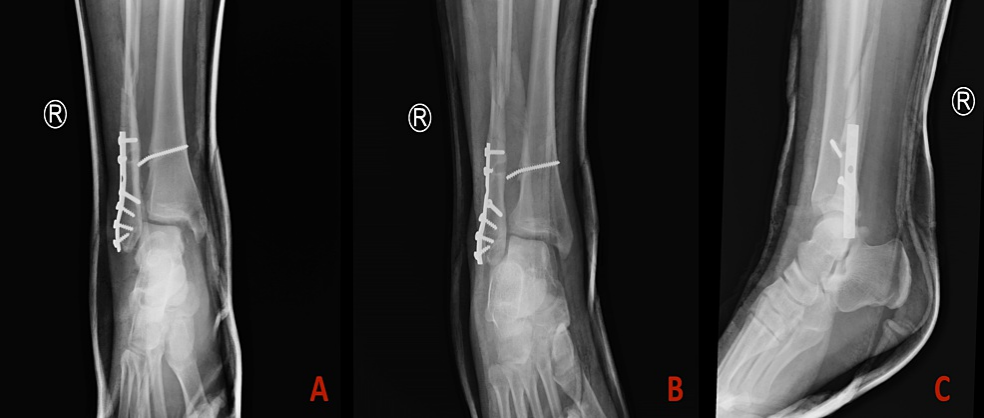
Preoperative radiographs from 05/04/2023 depict fibular fracture and previous ORIF hardware. A: Anterior-posterior view. B: Oblique view. C: Lateral view ORIF: open reduction and internal fixation
